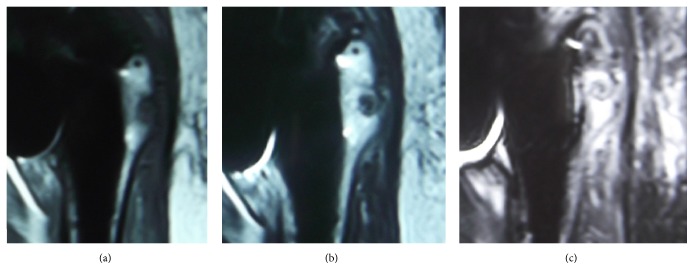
Magnetic resonance imaging at 10 weeks after THA. (a) T1-weighted image. (b) T2 star-weighted image. (c) T2 Short-TI Inversion Recovery (STIR) image.
